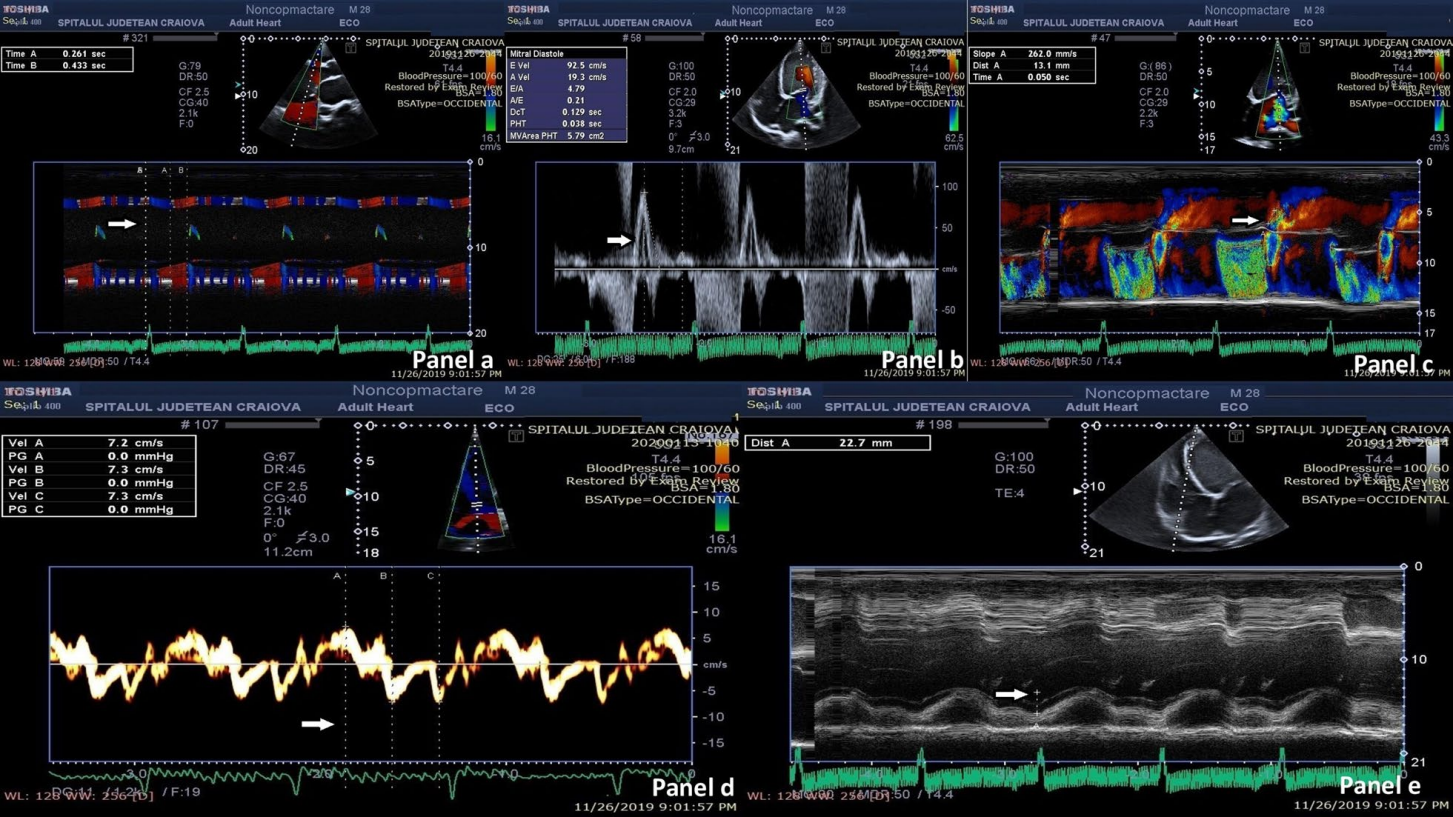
a Color M-mode; septal to posterior wall motion delay (SAPWD) calculation: 140ms. b Pulsed wave (PW) Doppler of mitral diastolic inflow, revealing a restrictive filling Pattern. c Color M-mode al the level of the mitral valve with velocity propagation (vp) measurement. d 2D tissue Doppler echocardiography with e wave velocity measurement. e M-mode echocardiography at the level of lateral tricuspid annulus providing tricuspid annular plane systolic excursion (TAPSE)
